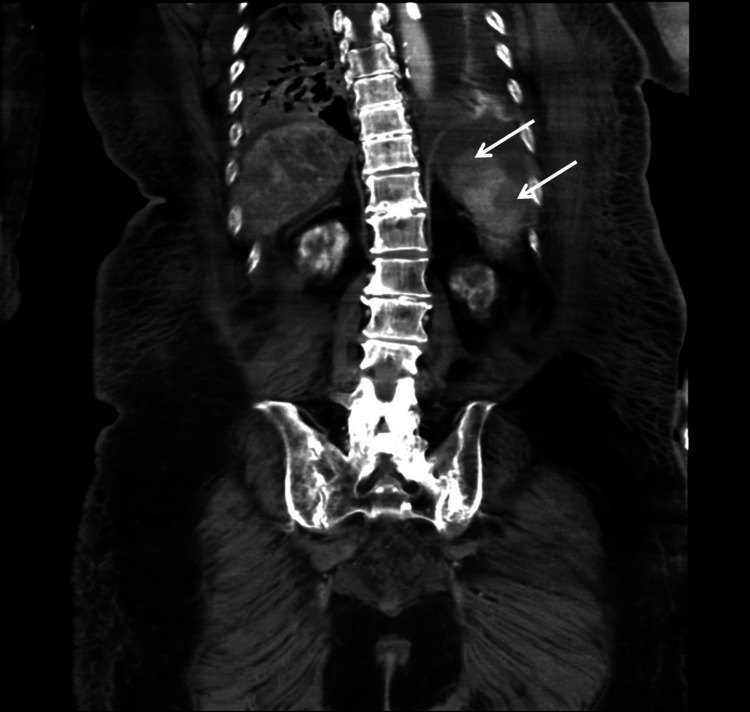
Contrast-enhanced CT, coronal section of the spleen Contrast-enhanced computed tomography (CT) showed regions of non-enhancement in the spleen (white arrows).
